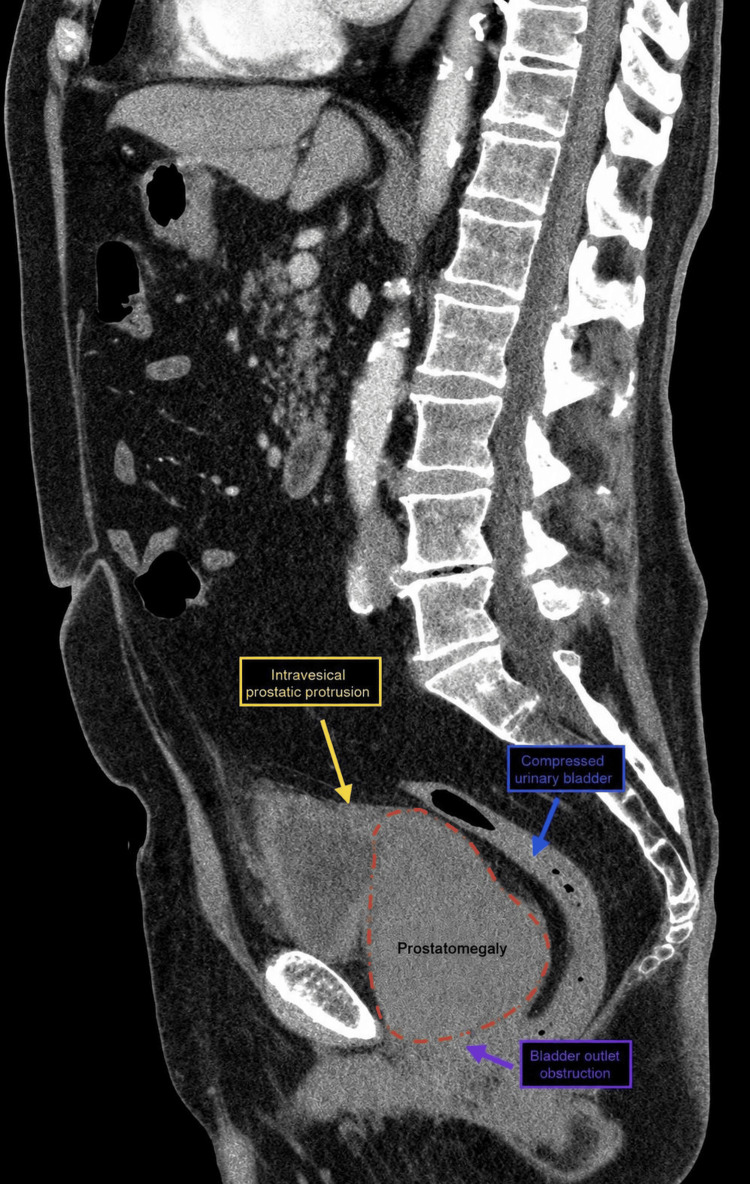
Sagittal contrast-enhanced CT Image demonstrating marked prostatomegaly with significant intravesical prostatic protrusion causing bladder outlet obstruction
